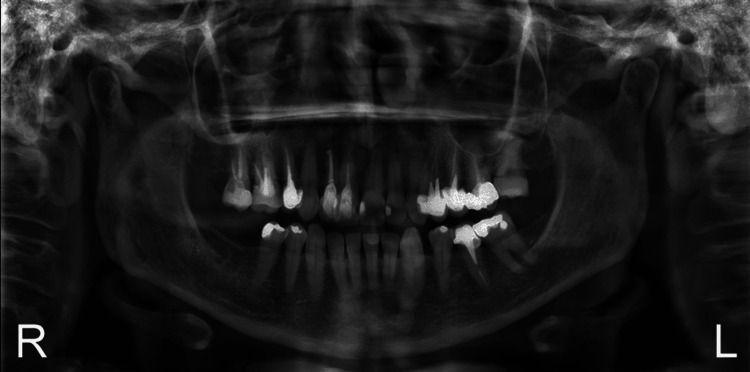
Initial panoramic radiograph demonstrating absence of teeth #46 and #47, adequate bone for implant placement, and no periapical pathology
